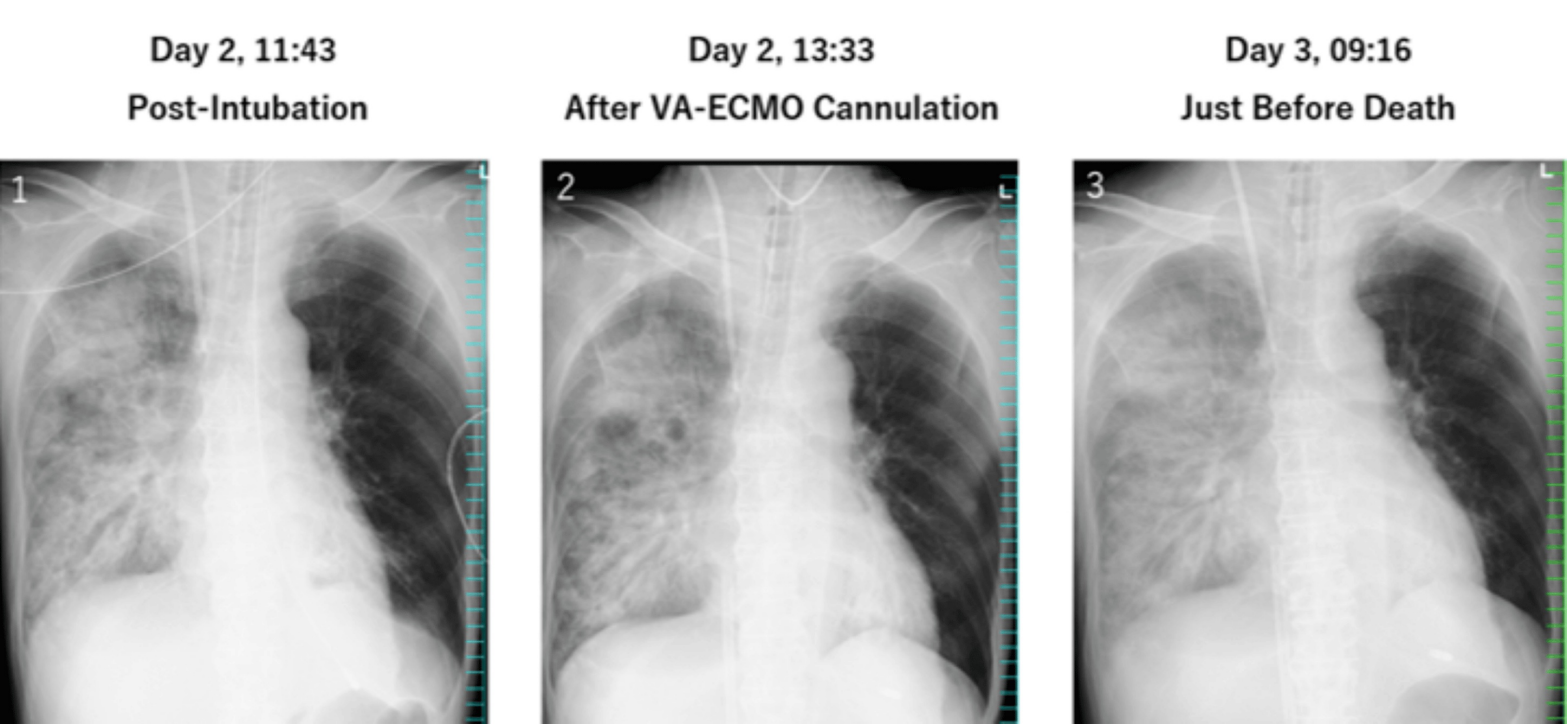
Sequential chest X-ray changes and rapid progression of unilateral pulmonary consolidation (Slide 1) Day 2 at 11:43 a.m., post-intubation: chest X-ray after endotracheal intubation. Initial unilateral pulmonary consolidation was noted. (Slide 2) Day 2 at 13:33 p.m., after VA-ECMO cannulation: chest X-ray after veno-arterial extracorporeal membrane oxygenation (VA-ECMO) initiation. Increasing bronchial wall thickening was noted. (Slide 3) Day 3 at 09:16 a.m., just before death: final chest X-ray taken shortly before death. Marked bronchial dilatation consistent with necrotizing pneumonia.
